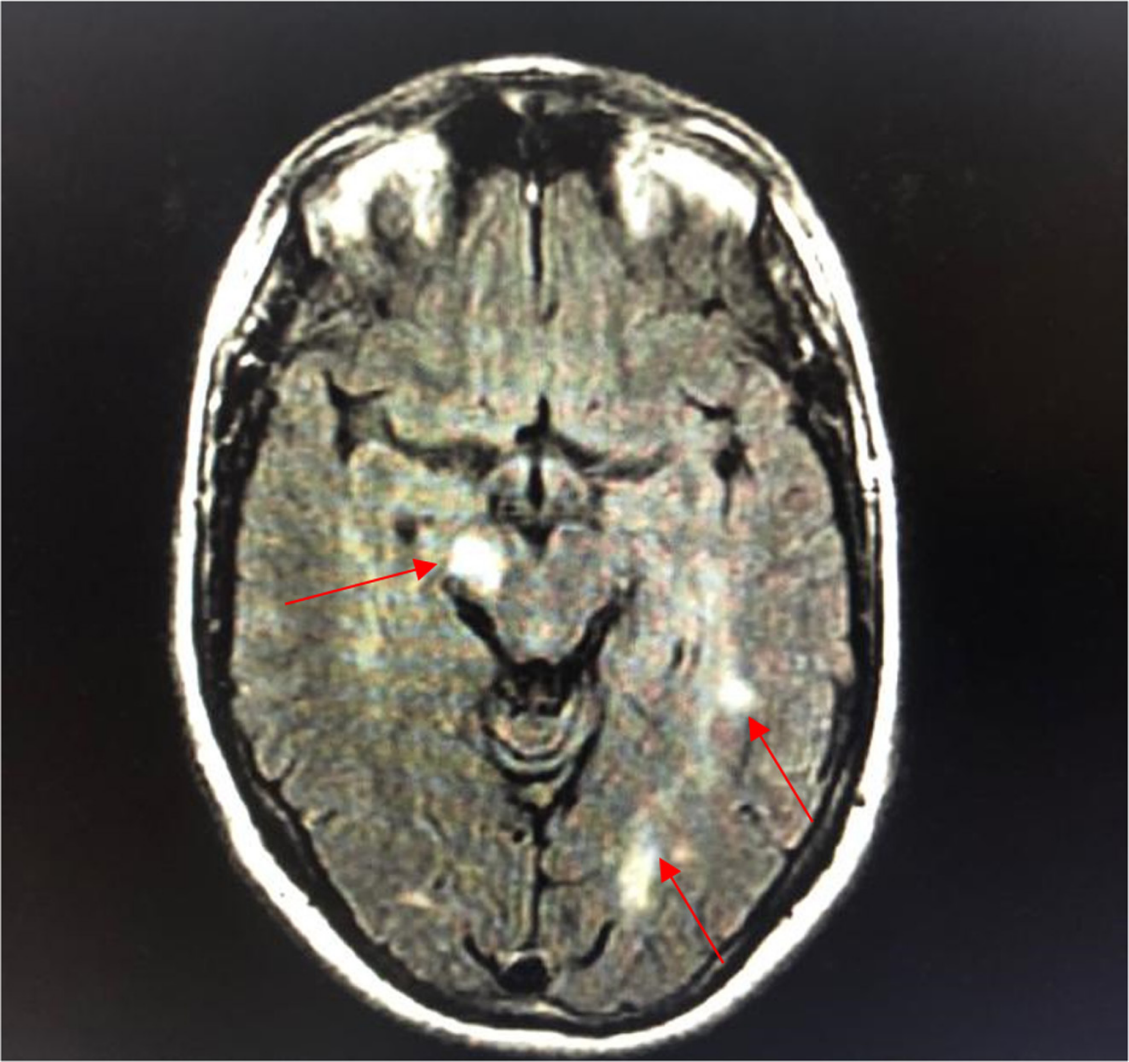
T2 FLAIR MRI of the brain in August year X showing signal abnormalities of the right midbrain and left temporal lobe.
